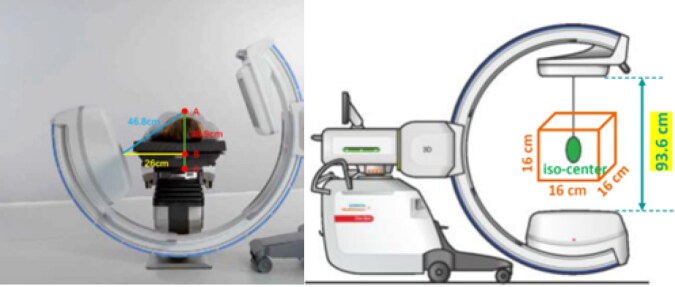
System Limitations and Patient Adaptations. Left panel: The square of the length of line segment AB must not exceed the difference between the square of the maximum diameter of the Cios Spin CT arm entrance and half the width of the carbon bed squared (see Figure 3). Right panel: Both the target lesion and the skin puncture site must be located within the 16 × 16 × 16 cm region centred at the iso-centre (see Figure 3). Abbreviation: CT, computed tomography.
